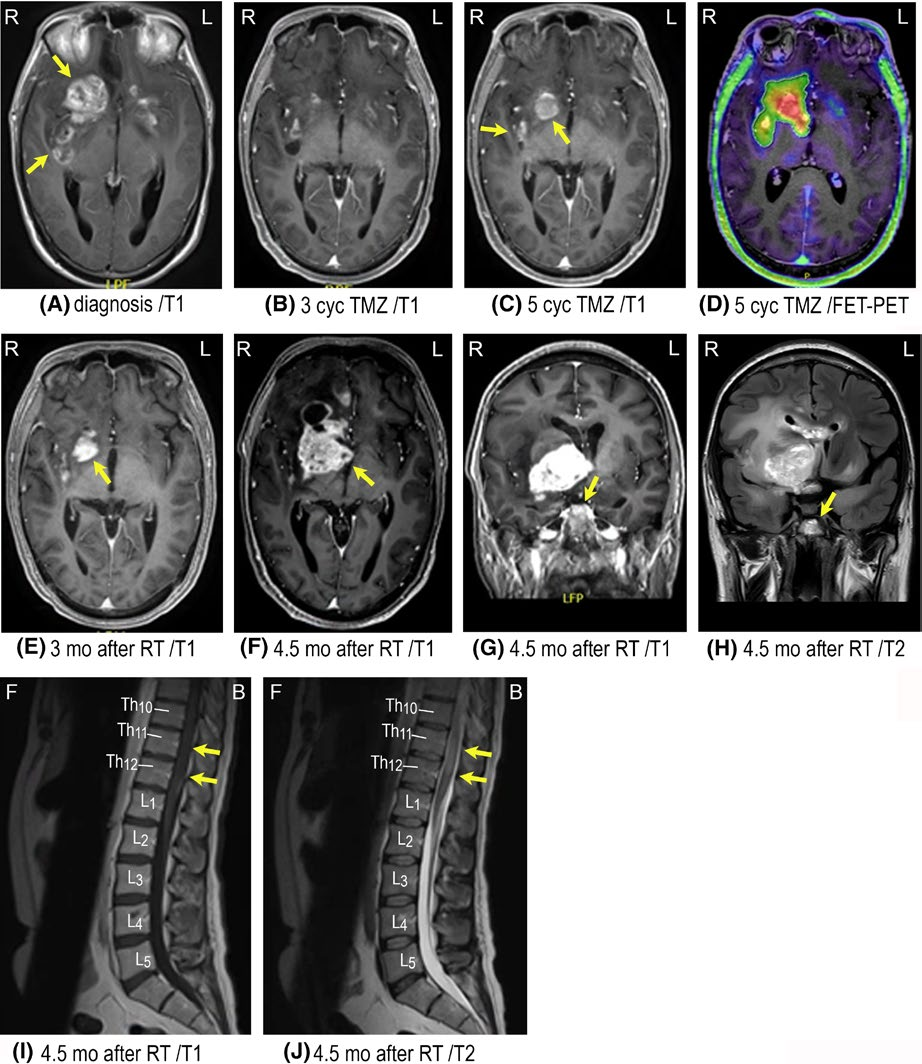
Tumor imaging of Case 1. A, Preoperative T1‐weighted MRI with a 3.3 × 3.6 cm large contrast‐enhanced (CE) tumor involving genu corpus callosum, both frontal lobes, right temporal lobe and both basal nuclei including subependymal lesions in the left frontal horn. B, T1‐weighted MRI after three series of temozolomide (TMZ), showing regression. C, T1‐weighted MRI after five series of TMZ with suspicion of progressive disease (PD). D, FET/PET with confirmation of PD with increased metabolic activity in the tumor. E, T1‐weighted MRI of tumor regression after radiotherapy. F‐H, PD in all lesions including spread to the fourth ventricle shown on both T1 and T2‐FLAIR MRI. I‐J, Non‐CE tumor in the spinal cord at levels TH11/Th12 on T1‐ and T2‐weighted MRI. Body orientation is indicated by R (right), L (left), F (front), and B (back)
